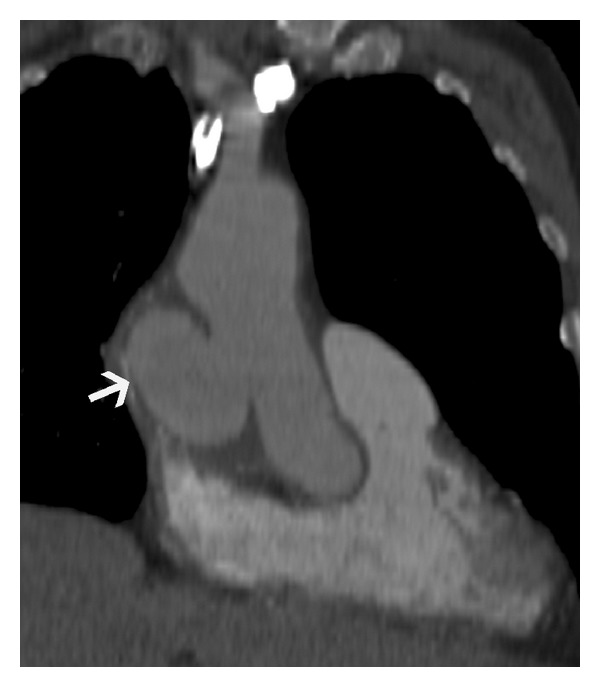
Pseudoaneurysm—coronal reconstructed CT image in a 53-year-old male following aortic surgery shows a pseudoaneurysm with narrow neck originating from the lateral aspect of the ascending aorta.
